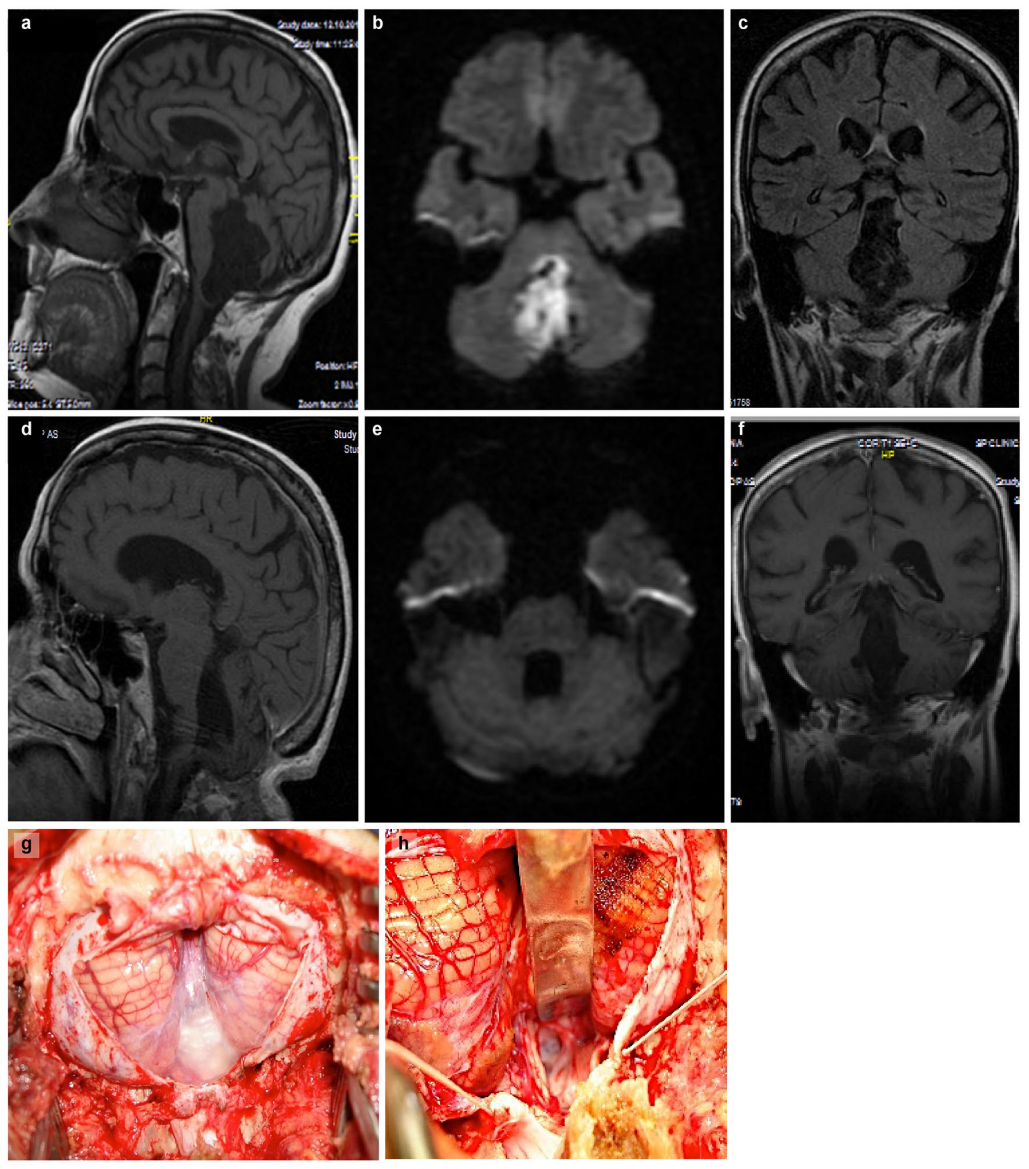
Patient 1, IVth ventricle epidermoid cyst: images (a–c)—preoperative MRI (T1 DWI in sagittal, axial and coronal sequences) revealed a hypodense mass, respectively hyperdense in DWI, filling the fourth ventricle; 3-month follow-up MRI: images (d–f) (T1 and DWI sequences) demonstrate gross-total resection without remanence at the level of the fourth ventricle floor; images (g) and (h)—intraoperative aspect, median suboccipital approach.
